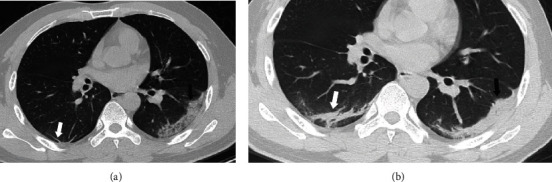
A 44-year-old man presented with fever, vomiting, nausea, and fatigue for 7 days. Axial CT image (a) shows patchy ground-glass opacities with focal consolidation (black arrow) in the left lower lobe. Parenchymal band is also manifested (white arrow) in the right lower lobe. Three days later, the second CT scan (b) shows increased attenuation of the lesion in the left lower lobe (black arrow) and thickening of the lesion in the right lower lobe (white arrow).
